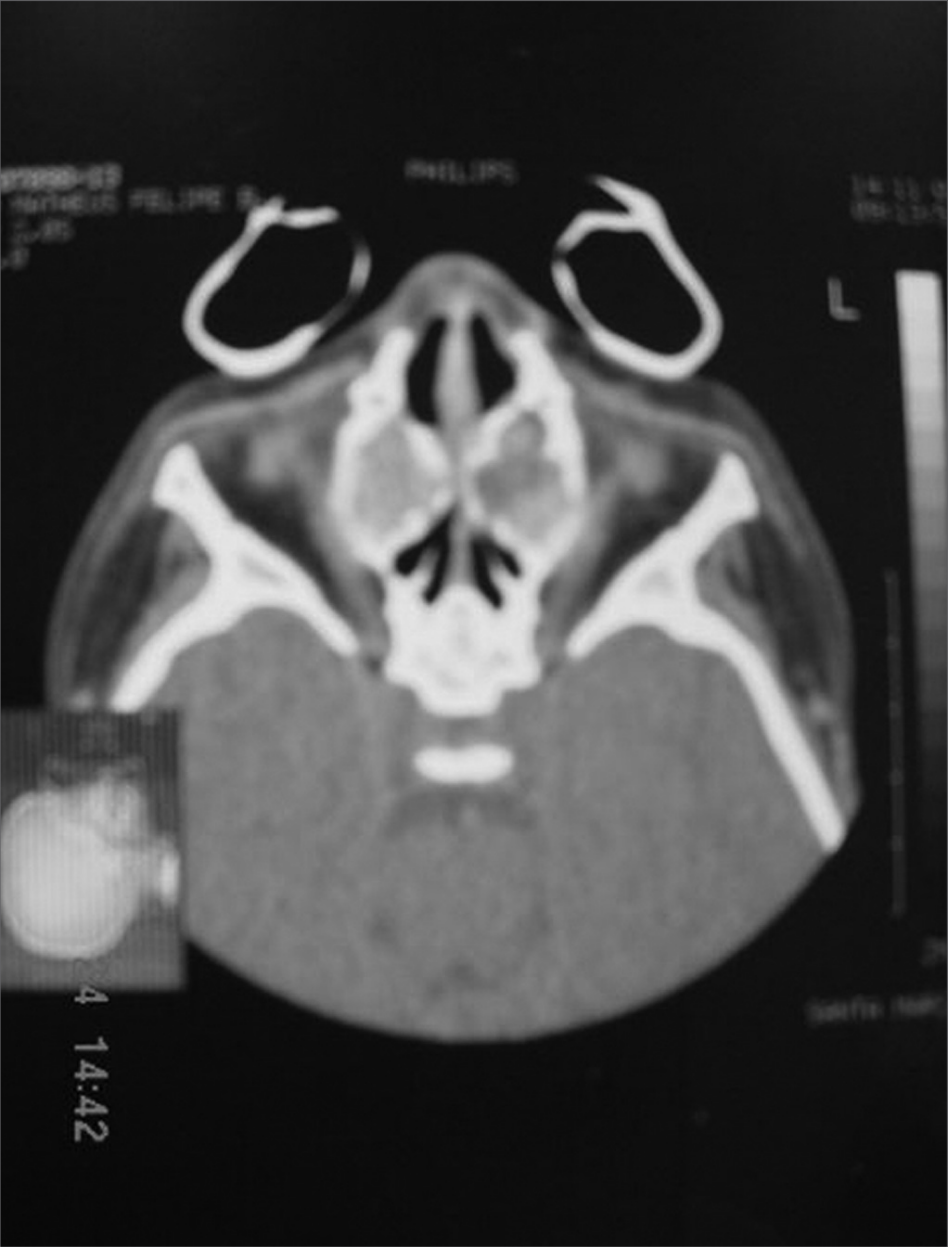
Paranasal sinuses CT Scan, axial view, bone window, showing nasal cavities obstruction caused by a bilateral maxillary cystic formation and a hypertrophy of pharyngeal tonsils.
